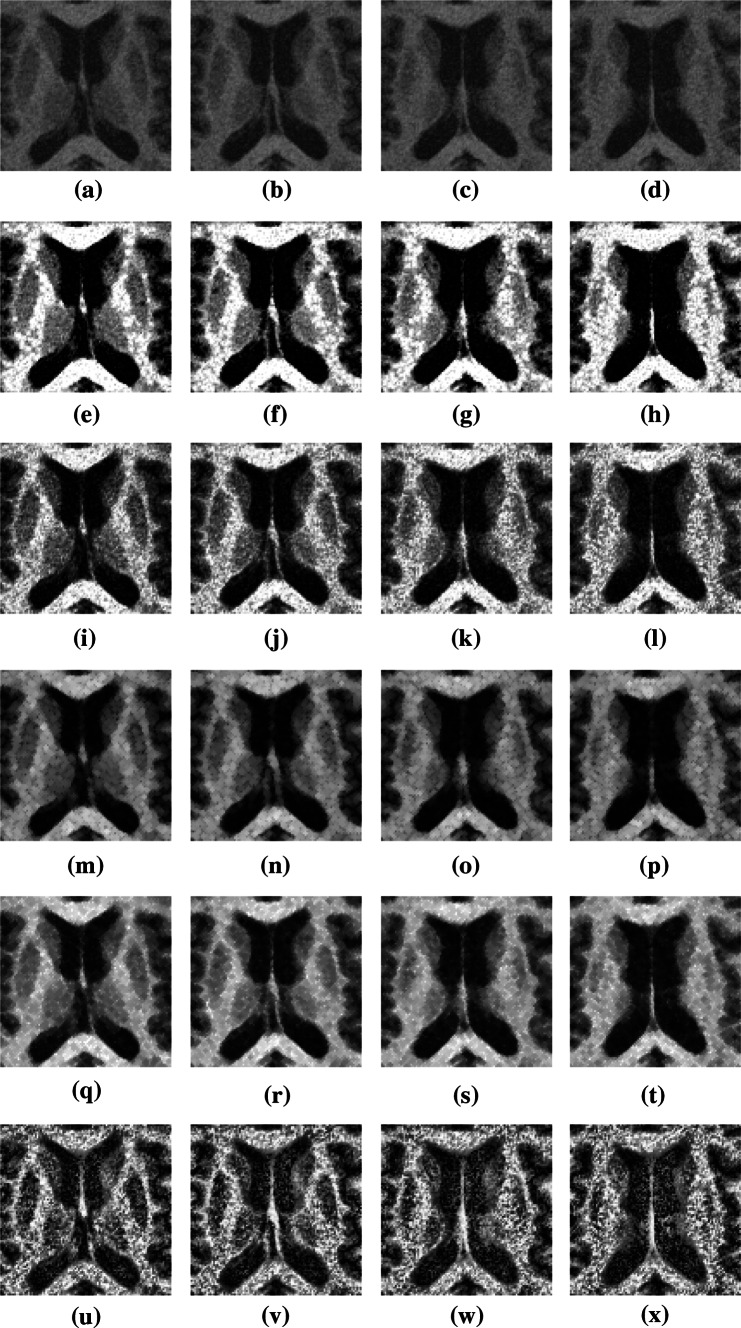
a–d MRI images of patient 2, e–h results obtained using proposed method, i–l HE images, m–p opening images, q–t closing images, and u–x CLAHE images
